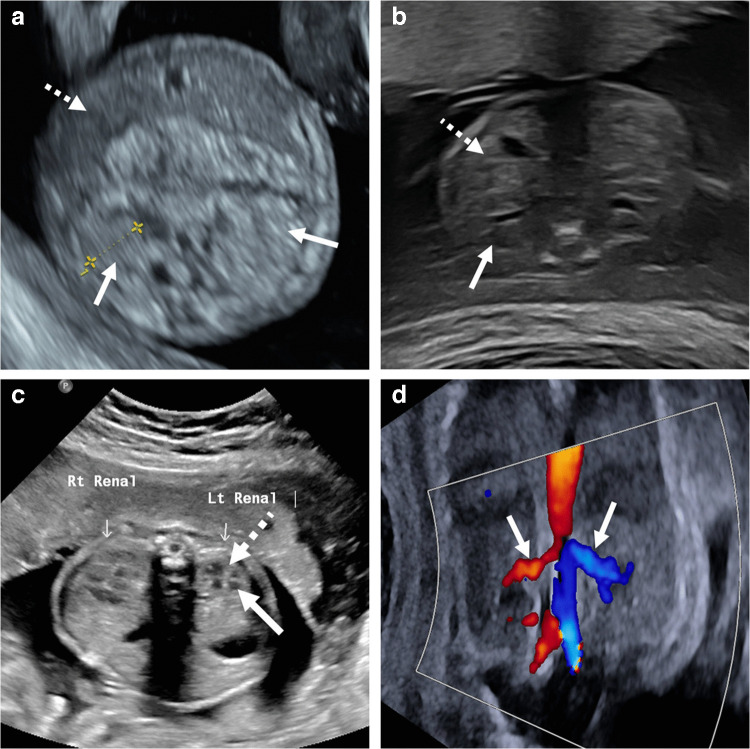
Fetal ultrasound of the kidneys with progressing gestation. a Axial ultrasound of a 15-week gestation male fetus showing that the renal parenchyma (*arrow*) is echogenic compared to the liver (*dotted arrow*). b Axial ultrasound of a 20-week gestation male fetus showing that the renal parenchyma (*arrow*) is now hypoechoic compared to the liver (*dotted arrow*). c Axial ultrasound of a 28-week gestation male fetus showing that the renal medulla (*arrow*) decreases in echogenicity becoming hypoechoic compared to the cortex (*dotted arrow*). d Coronal Doppler ultrasound of a 28-week gestation male fetus showing the renal arteries (*arrows*)
